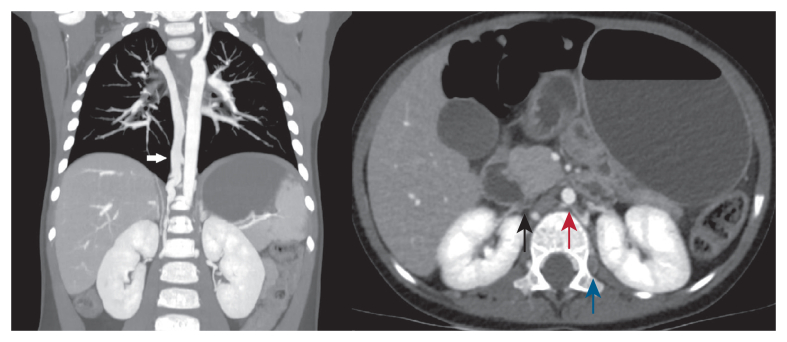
Computed tomography scan showing (A) the atretic inferior vena cava (black arrow), aorta (red arrow), and thrombosed lumbar vein (blue arrow) and (B) the dilated azygos system (white arrow).
